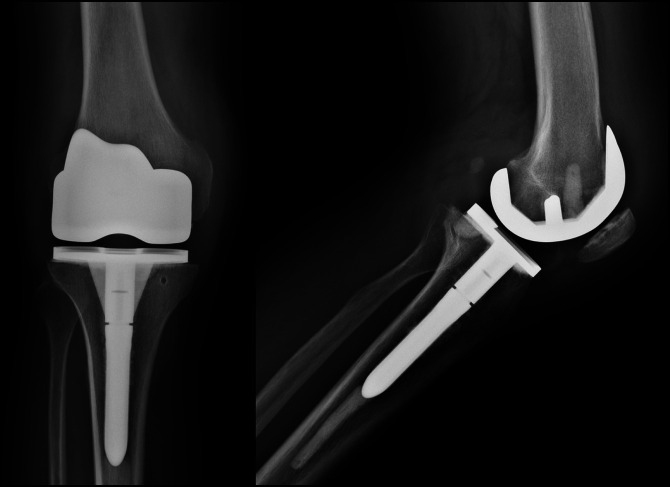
AP (left) and lateral (right) radiographs showing the right knee 3 months after index total knee arthroplasty.
